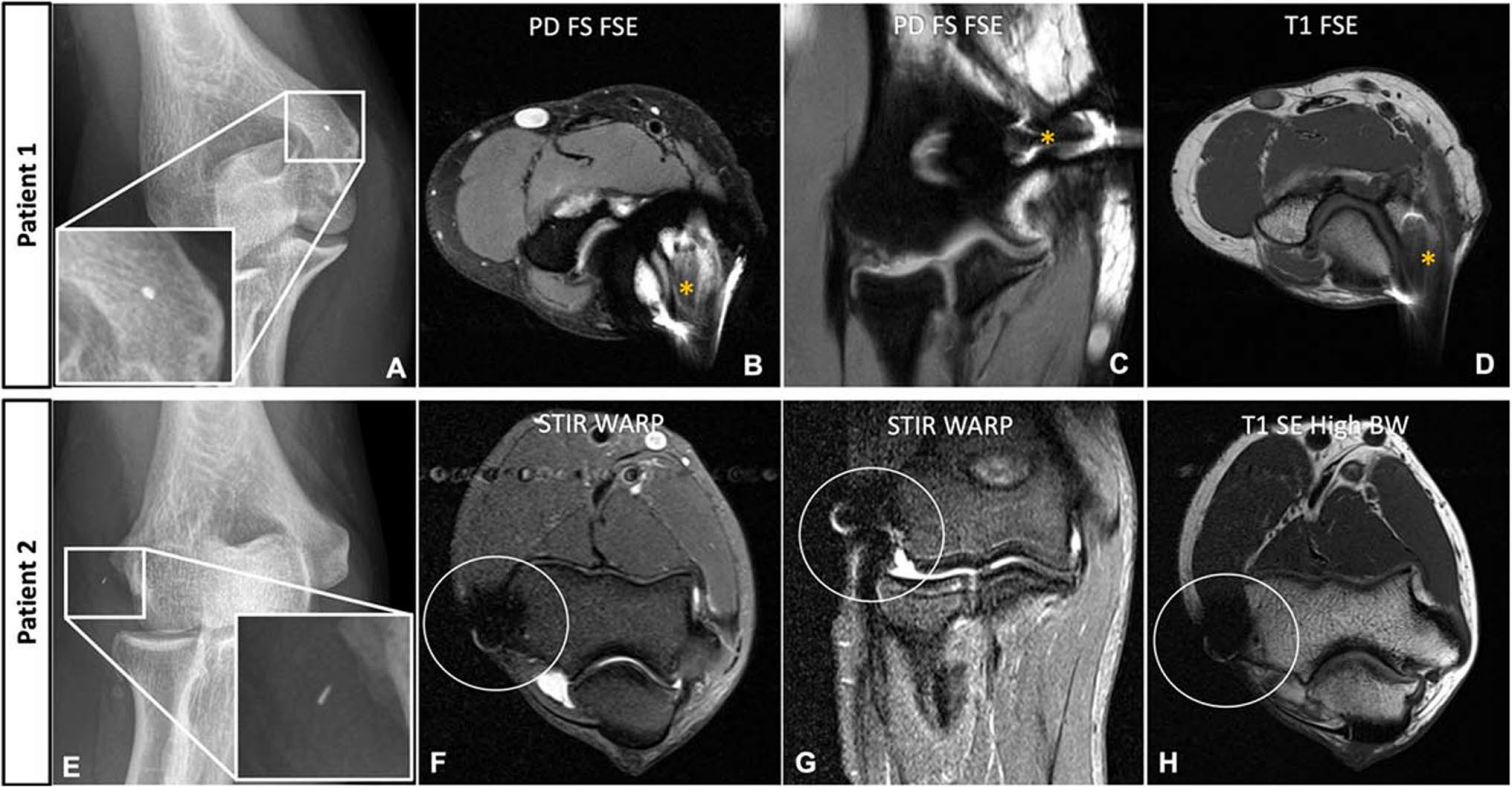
Signal pile-up and geometric distortion caused by a residual metal fragment after removal of orthopedic hardware from the distal humerus in a 38-year-old patient (patient 1, A–D) and a 41-year-old patient (patient 2, E–H). The MRI of the first patient was acquired with standard proton density turbo spin echo (PD TSE) sequences with fat saturation in axial (B) and coronal (C) orientation, as well as a standard T1-weighted TSE sequence in axial orientation (D). Note the significantly increased signal pile-up and geometric distortion in the standard sequences (asterisks). Additionally, a failure of fat suppression can be seen on the coronal PD TSE sequence around the metal fragment (C). Patient 2 was examined with dedicated metal artifact reduction sequences including an axial and sagittal short-tau inversion recovery (STIR) TSE sequence with optimized inversion pulse (STIR WARP) (F, G) and a high bandwidth (BW) T1-weighted TSE sequence. Note that only localized artifacts are seen around the metal fragment (white circle). The severity of artifacts is due to the ferromagnetic properties of the broken-off metal fragments. The images were acquired at 1.5 T with following sequence parameters: transverse PD FS—echo time (TE) 26 ms, repetition time (TR) 2902 ms, receiver bandwidth 168 Hz/pixel; coronal PD FS—TE 24 ms, TR 2341 ms, receiver bandwidth 104 Hz/pixel; T1 FSE—TE 11 ms, TR 603 ms, receiver bandwidth 107 Hz/pixel; transverse STIR WARP—TE 41 ms, TR 3000 ms, receiver bandwidth 360 Hz/pixel, inversion time (TI) 130 ms; coronal STIR WARP—TE 22 ms, TR 3000 ms, receiver bandwidth 400 Hz/pixel, IT 130 ms; T1 SE High BW—TE 11 ms, TR 400 ms, receiver bandwidth 270 Hz/pixel
